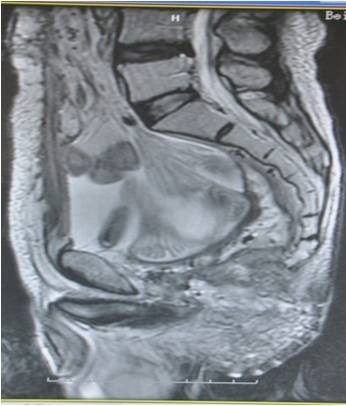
Sagittal section of magnetic resonance image after operation.
